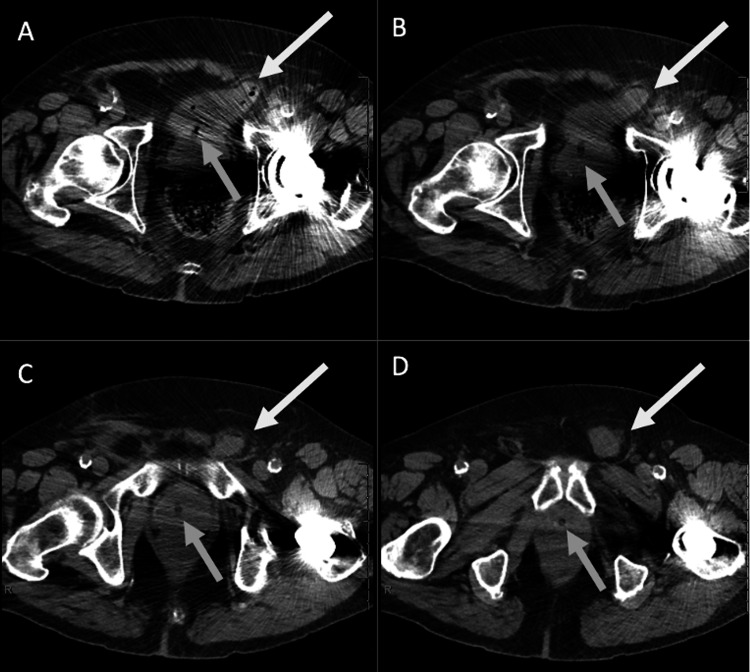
The axial non-contrast CT portion of a urogram as part of hematuria workup, progressing superiorly (A) through the level of the femoral heads (B & C), and inferiorly (D) to the lower pelvis shows a left anterior bladder bulge containing fluid and gas locules that extend into the left inguinal canal (white arrows) The Foley catheter can be faintly seen (gray arrow). A total left hip arthroplasty results in streak artifact across all images at these levels.
